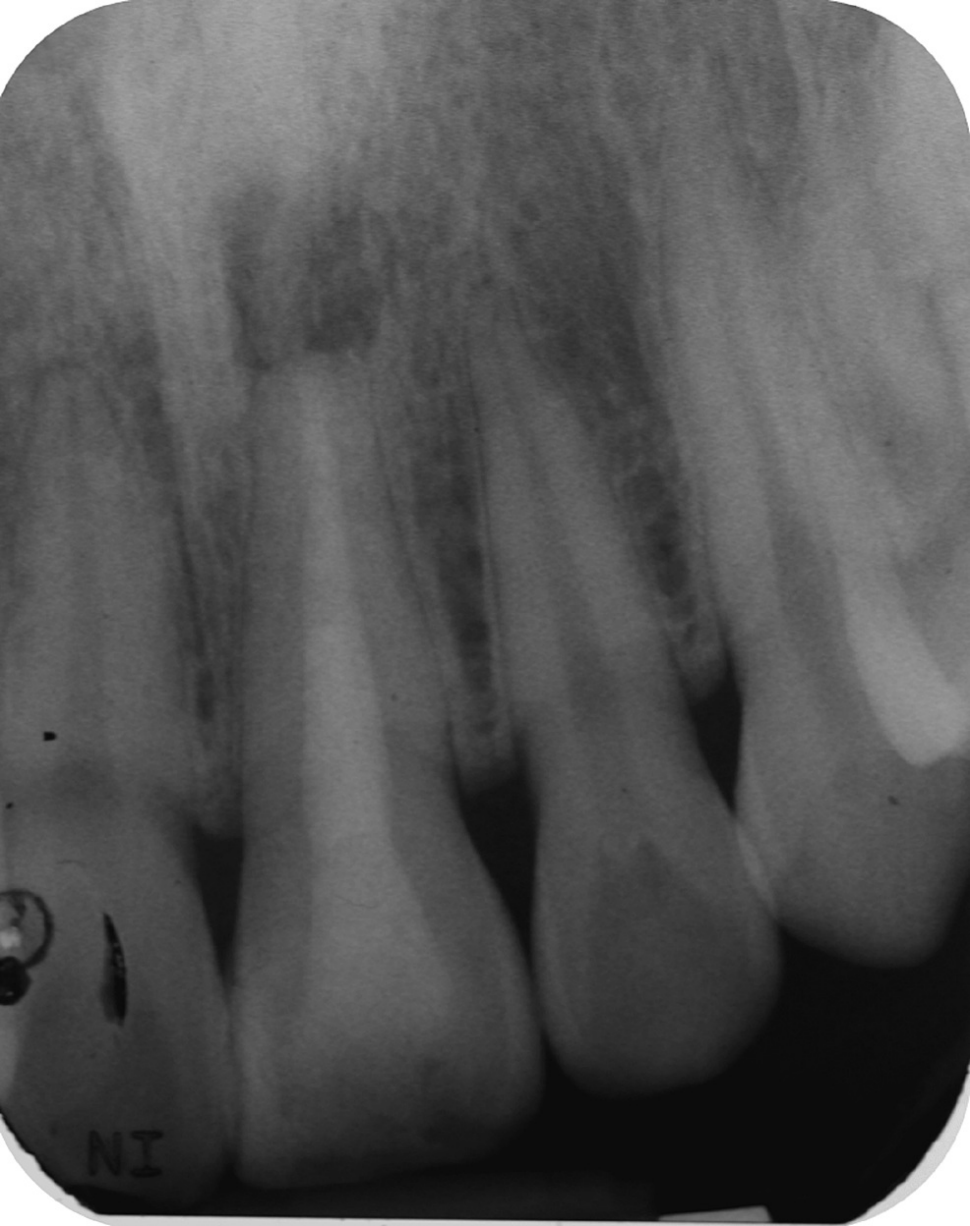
Radiograph after apical root resection
